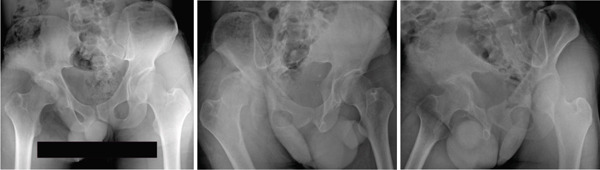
Case 5 preoperative radiographs.
